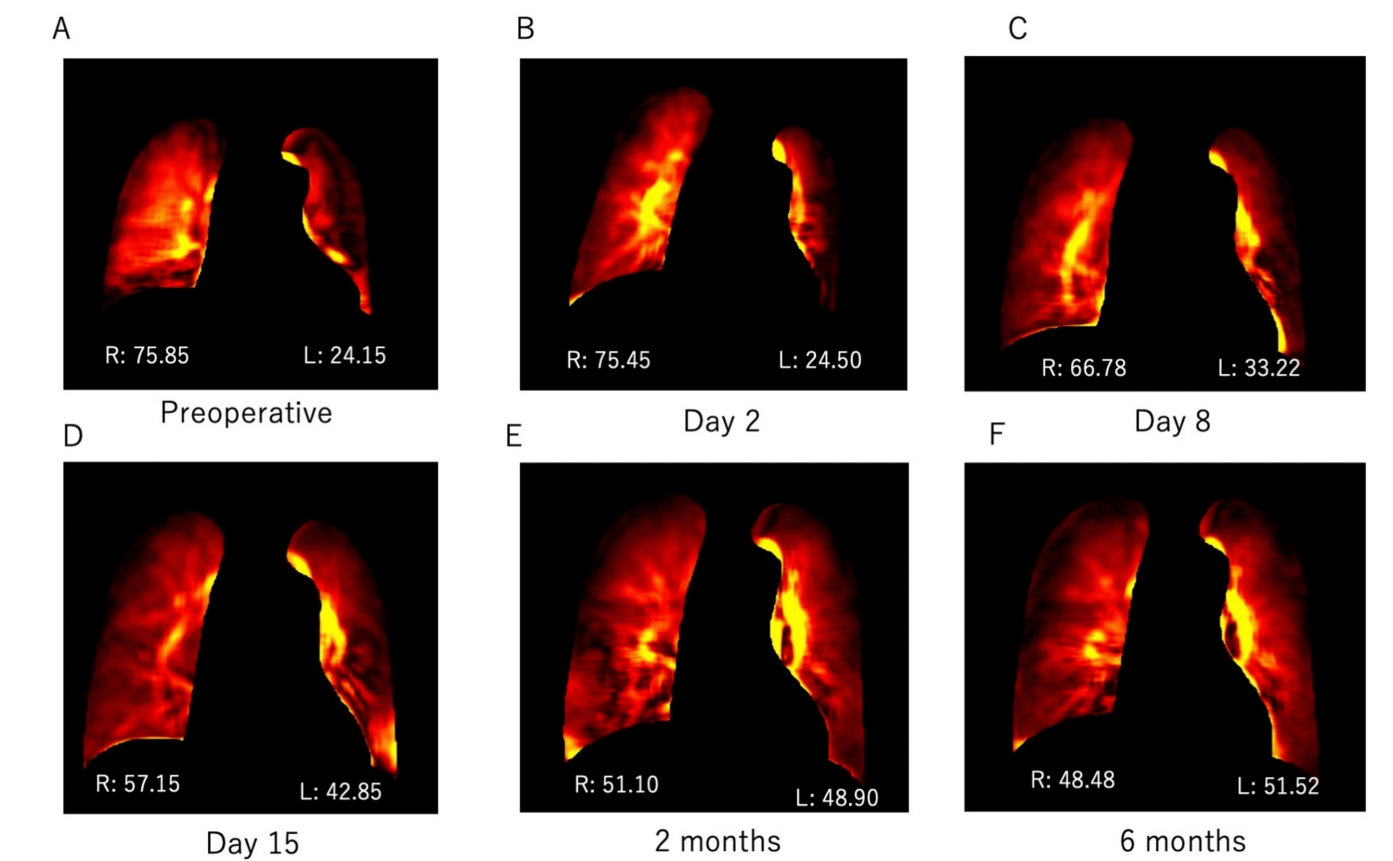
Pulmonary blood flow ratios between the transplanted right and left lungs in Case 2, pre-operatively and at different times post-surgery: dynamic digital radiography (DDR) results. A: Pre-operative, B: Day 2, C: Day 8, D: Day 15, E: 2 months, F: 6 months, R: right, L: left The number indicates the percentage, which is 100% including the left and right sides.
